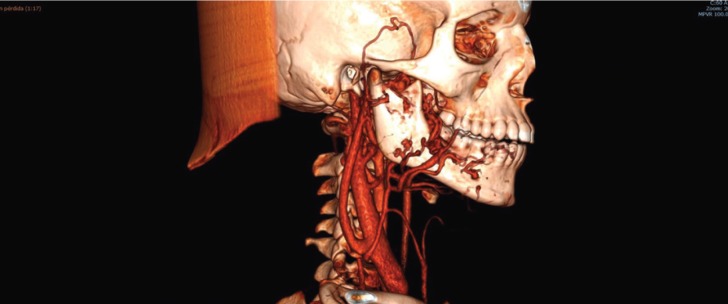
3D CT image of the arteriovenous malformation extending from the right mandibular body to the homolateral pterygoid region and infratemporal fossa.
